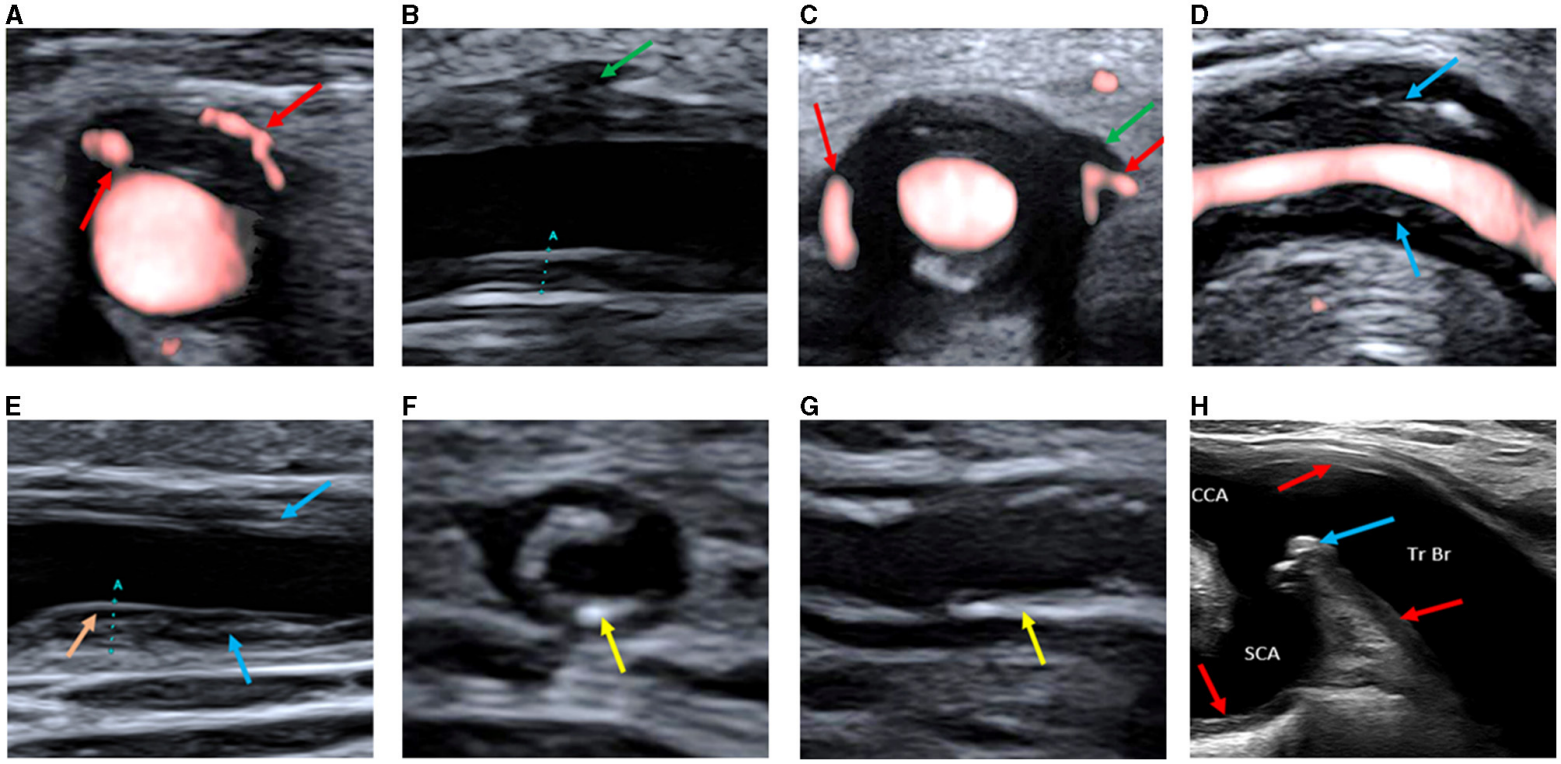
(A) Transverse ultrasound image of the common superficial temporal artery showing increased vessel wall thickness with hypoechogenicity and neovascularization in the wall (red arrows). (B) Longitudinal ultrasound image of the common superficial temporal artery showing a hypo-low-echogenic area outside the vessel wall (interpreted as inflammatory oedema, green arrow). The marker (A) is the IMT measurement. (C) Transverse ultrasound image of the common superficial temporal artery showing a hypo-low-echogenic area outside the vessel wall (interpreted as inflammatory oedema, green arrow) and neovascularization both in and outside the wall (red arrows). (D) Longitudinal ultrasound image of the facial artery showing increased vessel wall thickness with medium-echogenic areas (blue arrows), without neovascularization or hypo-echogenic areas outside the vessel wall. (E) Longitudinal ultrasound image of the common superficial temporal artery showing increased vessel wall thickness with medium-echogenic areas (blue arrows), without neovascularization or hypo-echogenic areas outside the vessel wall. A hypo-echogenic area interpreted as oedema below the intima layer is seen in the far wall (orange arrow). The marker (A) is the IMT measurement. (F) Transverse ultrasound image of the common superficial temporal artery showing increased vessel wall thickness with high-echogenicity areas (yellow arrows). (G) Longitudinal ultrasound image of the common superficial temporal artery showing increased vessel wall thickness with high-echogenicity areas (yellow arrow). (H) Ultrasound image of the brachiocephalic trunk and proximal parts of common carotid and subclavian artery, showing both inflammatory changes in the vessel walls (red arrows) and atherosclerotic plaque (blue arrow). SCA, subclavian artery; CCA, common carotid artery; Tr Br, brachiocephalic trunk; IMT; Intima-media thickness.
